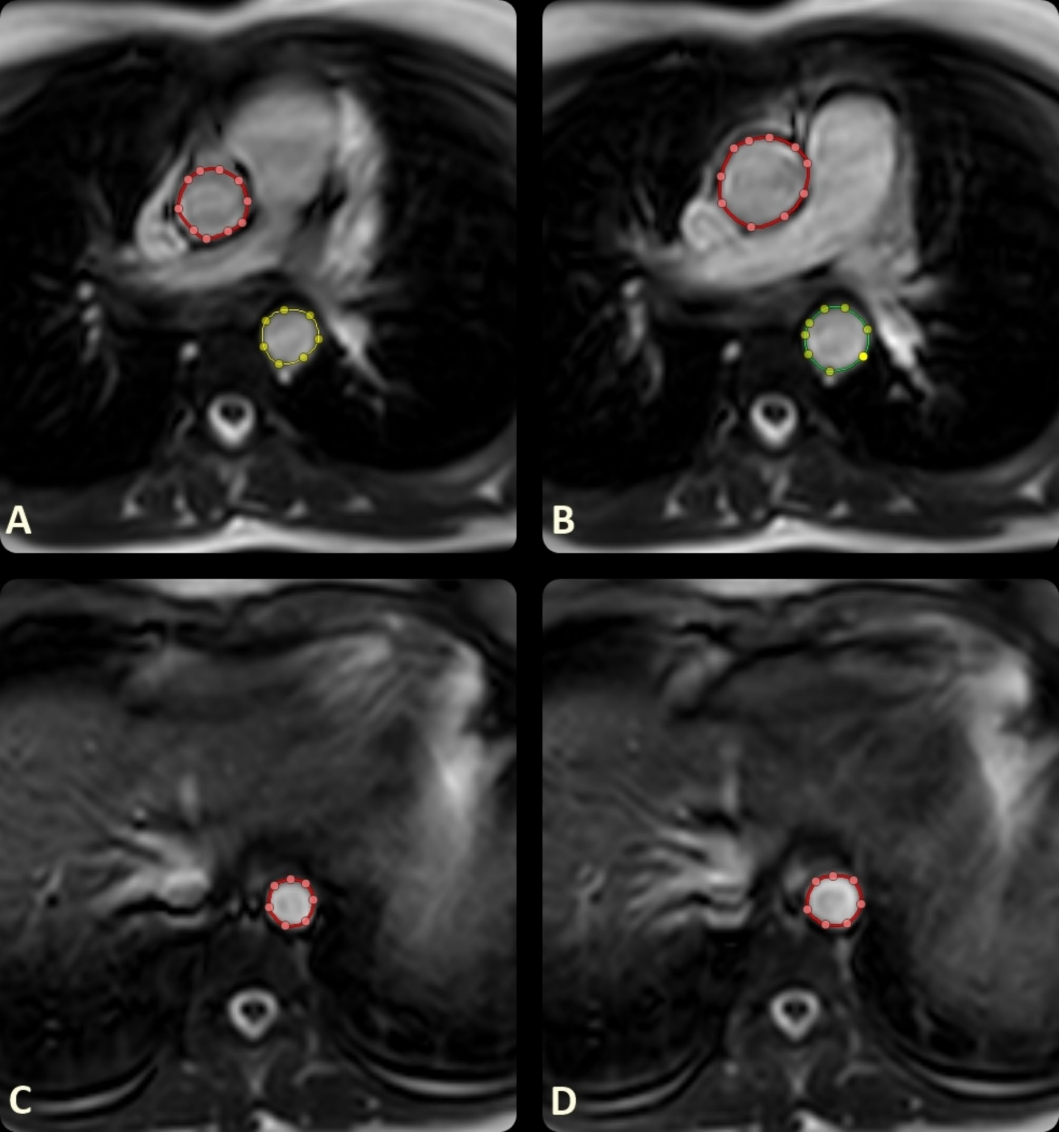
Cardiac MRI axial cine SSFP images show measurements of minimal and maximal cross sectional areas of ascending aorta and proximal descending aorta (A, B); and aorta at diaphragm (C, D).
